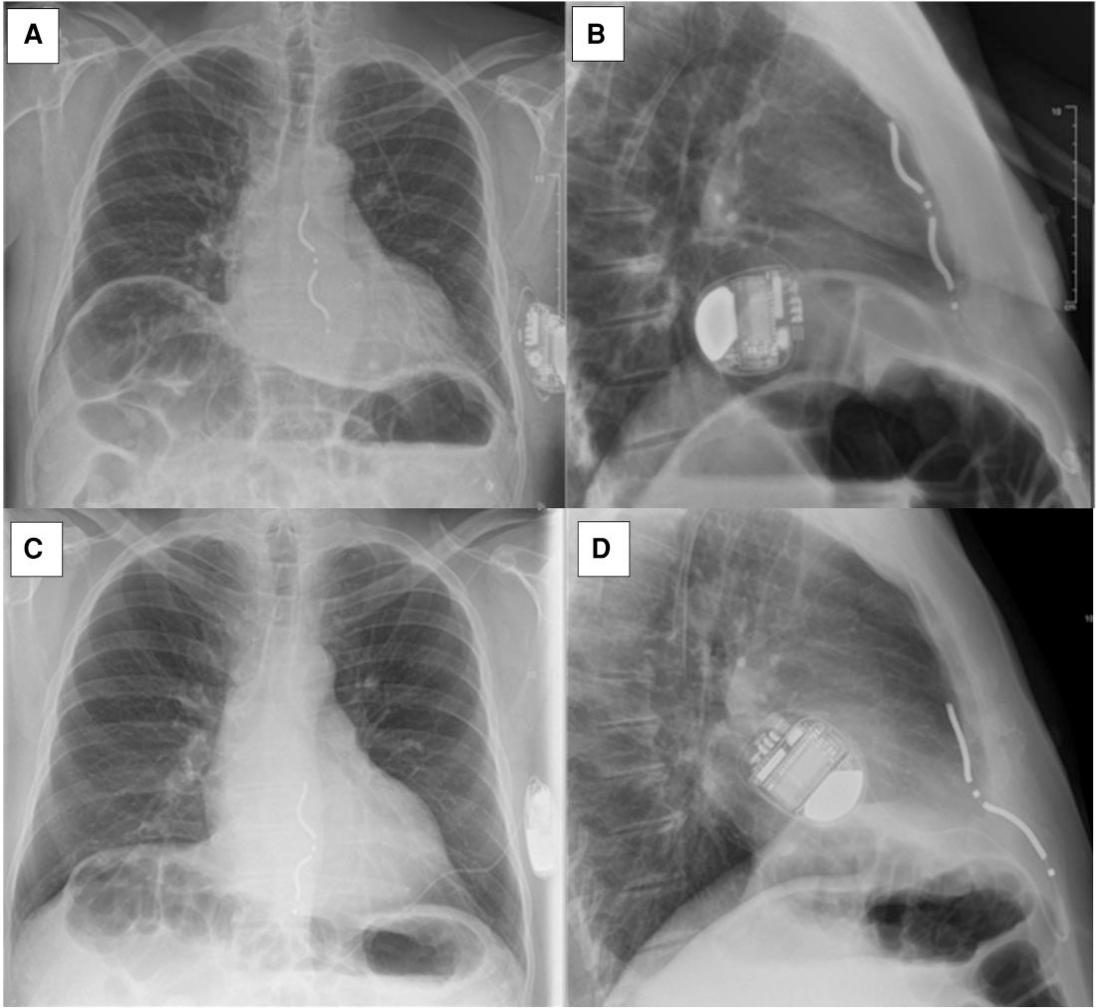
Posterior-anterior and lateral chest X-rays of extravascular implantable cardioverter-defibrillator. (*A*) Posterior-anterior and (*B*) lateral chest X-rays immediately post-implant. (*C*) Posterior-anterior and (*D*) lateral chest X-rays 3 months post demonstrating lead displacement and rotation of device of nearly 180 degrees with the lead coiled around it.
